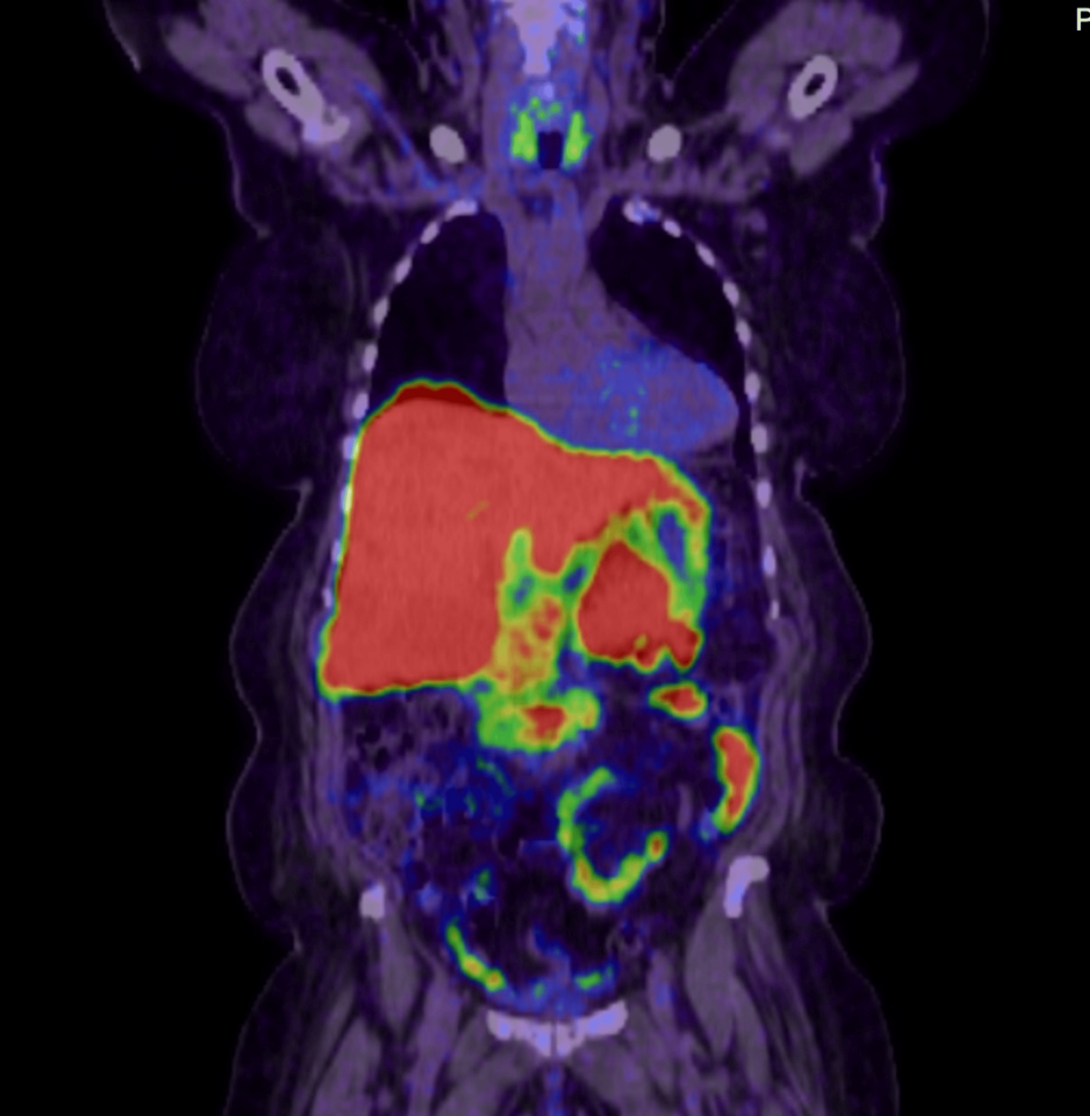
CT PET NETSPOT Approximate size of pancreatic mass is 5.9 × 3.3 cm. The largest mass in the liver is located in the inferior right hepatic lobe, measuring 5.6 × 5.6 cm. Somatostatin-positive pancreatic mass and numerous hepatic metastases. No additional sites of disease were identified.
